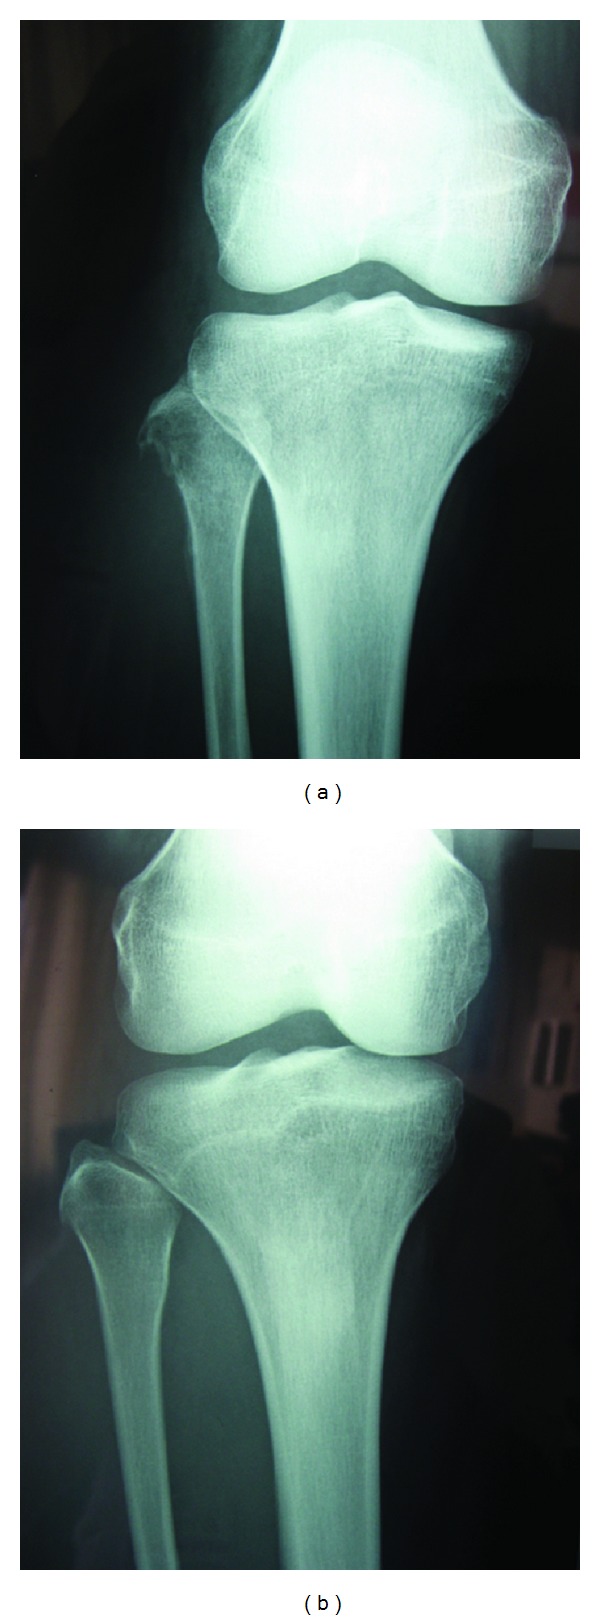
(a) 2 months later, the lytic lesion has rapidly progressed and destroyed the external cortical bone of the proximal peroneal head. (b) Initial right knee radiography where we notice a small bony spicule at the proximal fibula head.
